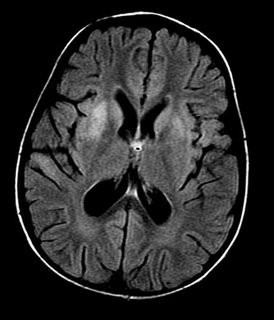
Magnetic resonance imaging at the time of admission showing increased signal intensity on T2 weighted images with symmetrical bilateral putaminal vasogenic edema with mild restriction on diffusion and no bleeding.
